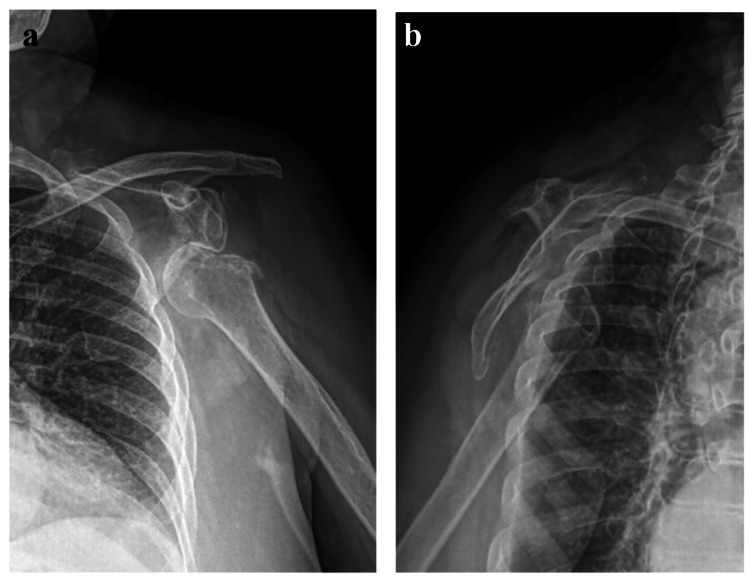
Pre-operative X-ray showing a subcoracoid subtype of anterior left shoulder dislocation showing an incongruent glenohumeral joint and an associated Hill-Sachs injury.
